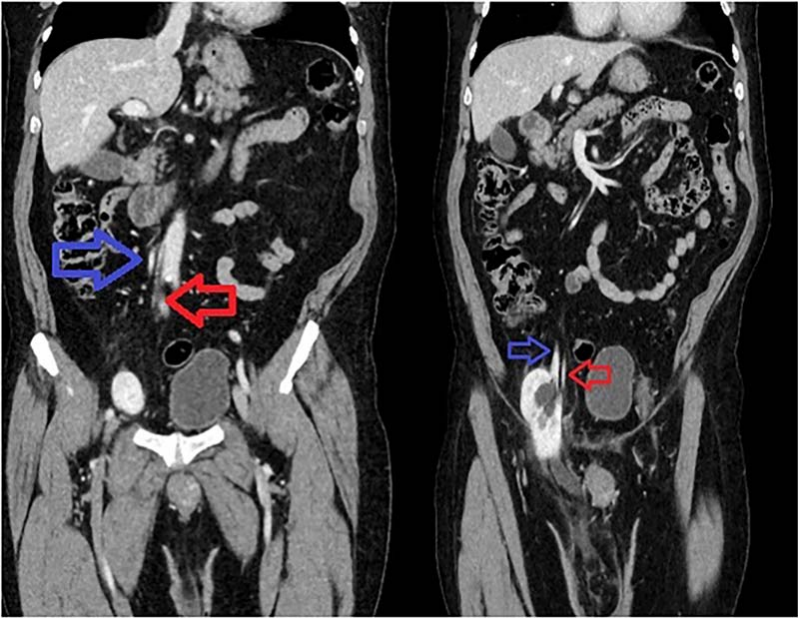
Sequential coronal CT with IV contrast. Left image showing renal artery (red) and vein (blue) arising from abdominal aorta and IVC. Right image showing descending vessels going into the incarcerated right kidney.
